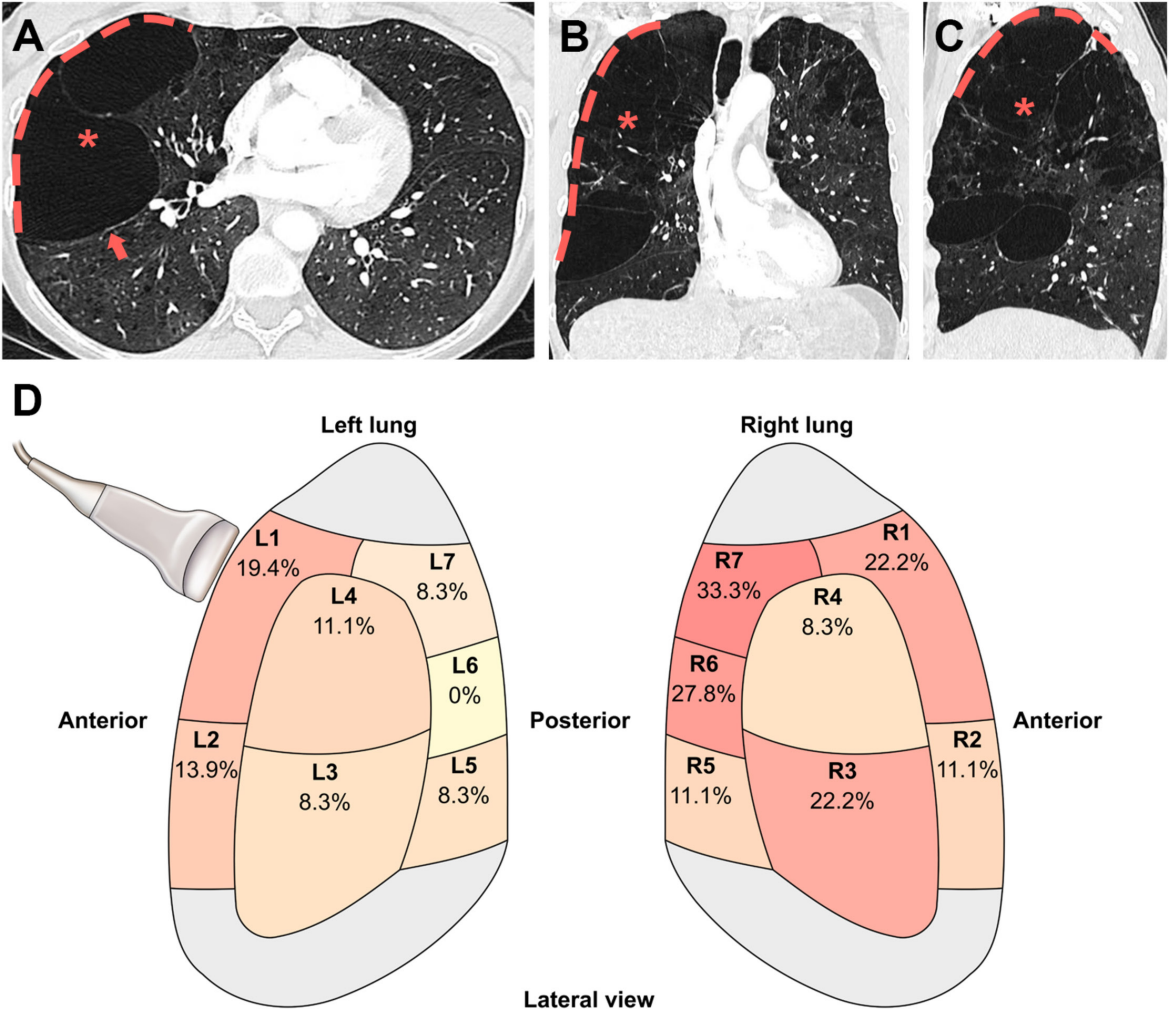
Bullous emphysema CT aspect and topography in the study population. (A–C) Representative chest CT sections (parenchymal window) of a voluminous bullous dystrophy (asterisk) with pleural contact (dotted line), with reconstruction in the axial (A), coronal (B) and sagittal (C) planes. Arrows indicate bullous wall, consisting of destroyed and compacted parenchyma. (D) Topography of the most voluminous bullae with pleural contact in the study population, according to CT scan. Each area is shown in lateral view on the surface of the right and left lungs (L1–L7 on the left, R1 to R7 on the right). Within each area, the percentage of included patients whose bullous emphysema with pleural contact involves that area is shown.
